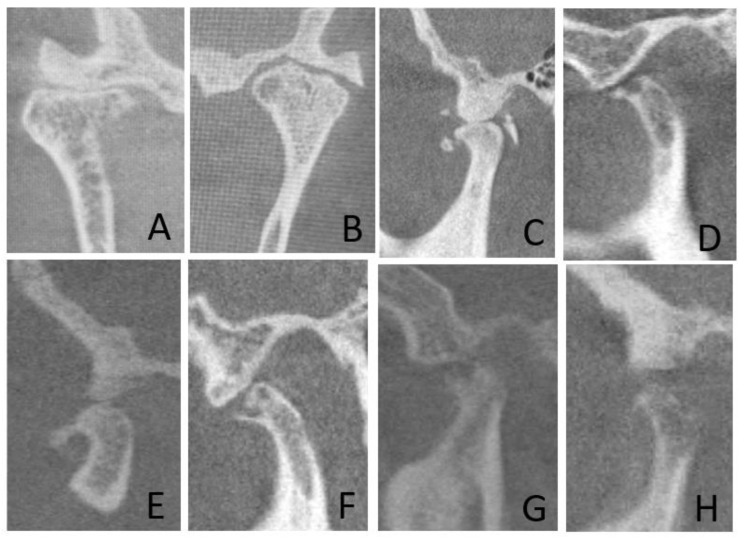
Examples of the CBCT images of osseous changes in the temporomandibular joints (TMJs). (A)—flattening of the condyle, subcortical sclerosis and ankylosis in a male with rheumatoid arthritis; (B)—joint space narrowing, subcortical cyst formation, condylar surface and articular eminence resorption in a female with scleroderma; (C)—loose calcified bodies in a male with rheumatoid arthritis; (D)—subcortical cyst formation in a female with psoriatic arthritis; (E)—flattening of the condyle and osteophyte formation in a male with osteoarthritis; (F)—multiple subcortical cysts in a female with rheumatoid arthritis; (G)—progressive condyle erosion in a male with pseudogout; (H)—articular eminence and condyle resorption in a female with SLE.
